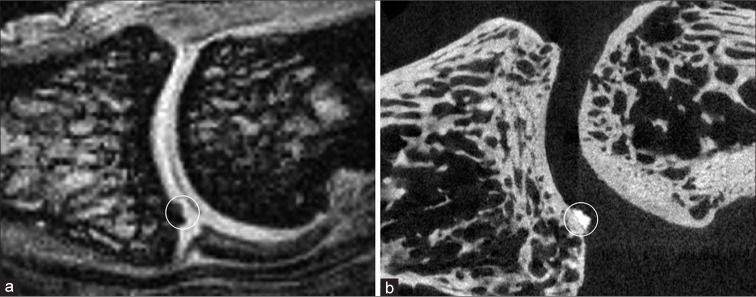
In this proximal interphalangeal joint, calcification inside the cartilage (circle) was observed on the sagittal (a) interleaved water-fat water + fat image as on the (b) microcomputed tomography image.
